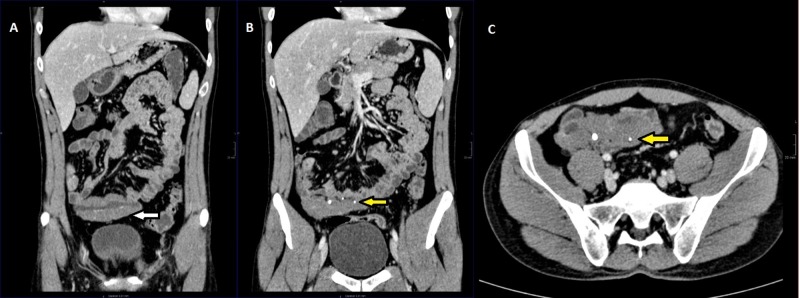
Two coronal and one axial reconstructions of computed tomography enterography. A, Coronal reconstruction shows the ileum mural thickening (white arrow). B, Coronal reconstruction depicts phleboliths (yellow arrow) and the ileum mural thickening. C, Same findings are showed on the axial reconstruction.
